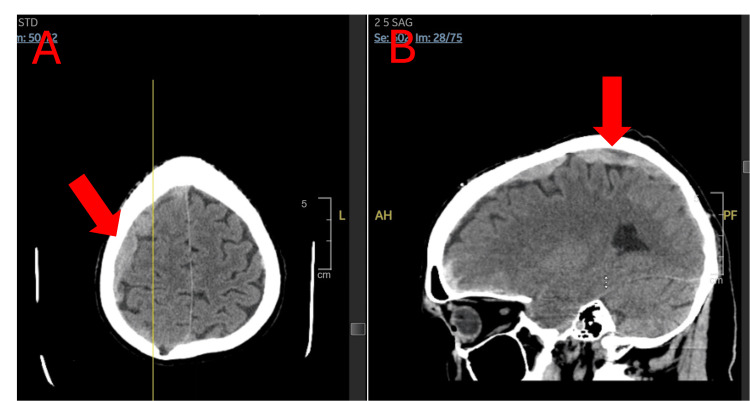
Initial CT of brain without contrast on admission displaying an anterior cranial fossa, suprasellar, bilateral sylvian fissure, and prepontine cistern subarachnoid hemorrhage in (a) transverse and (b) sagittal views.
